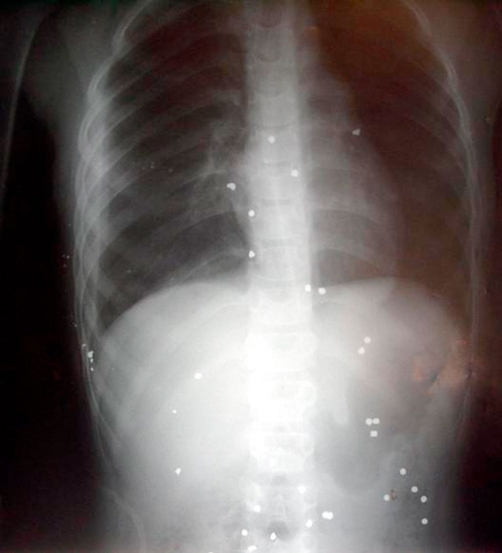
X-ray shows the pellets in the thorax and abdomen.
